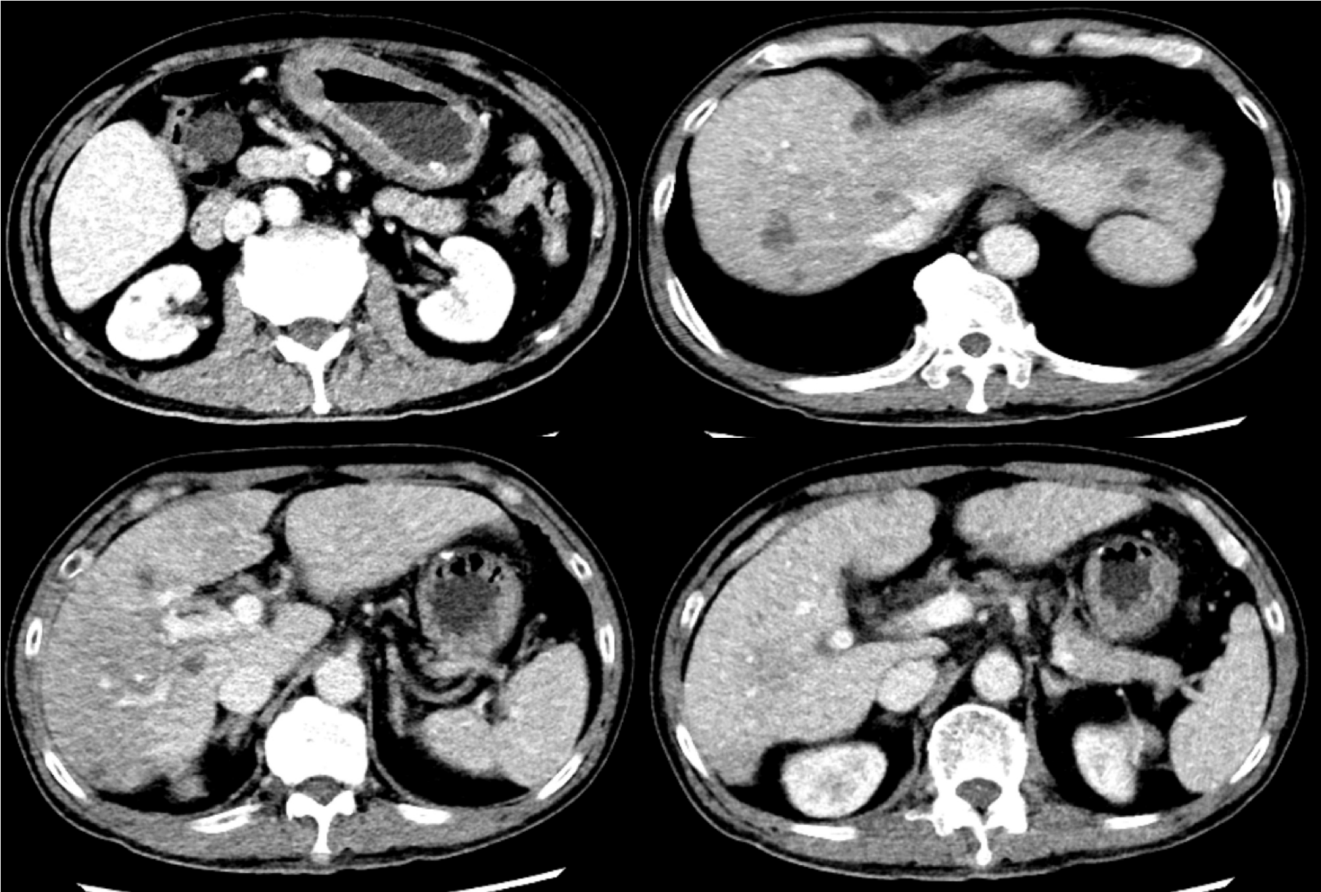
Partial response (PR) was achieved after 4 cycles of PRaG therapy, with continued tumor shrinkage on CT imaging.
